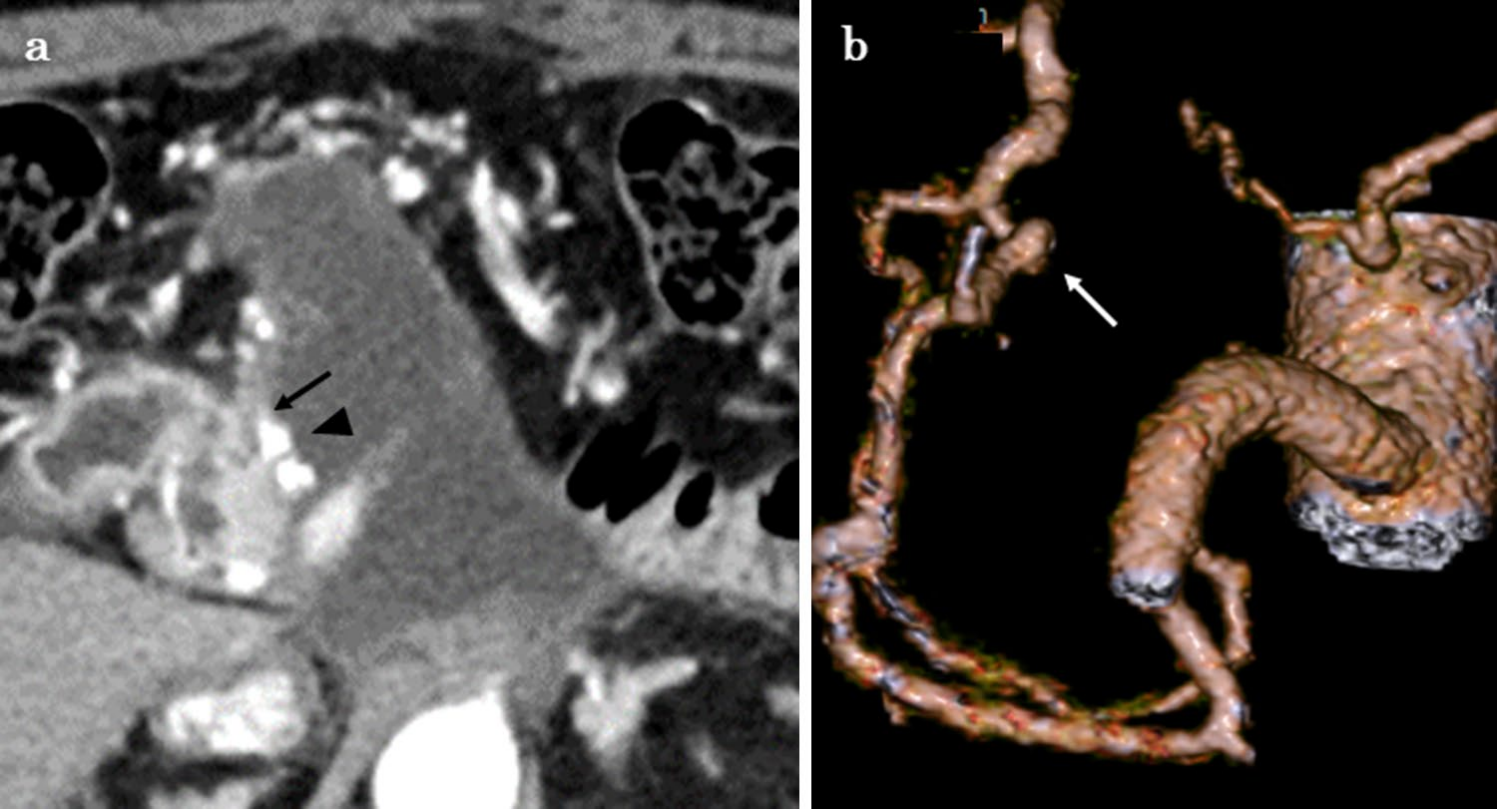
Enhanced CT findings three months after surgery. a: Enhanced CT image revealed a pseudoaneurysm (black arrowhead), 3 mm in diameter, arising from ASPDA (black arrow). Fluid collection was also observed near the pancreatic stump. b: Three-dimensional reconstruction image of the blood vessels revealed an aneurysm in the ASPDA (white arrow). *ASPDA* anterior superior pancreaticoduodenal artery, *CT* computed tomography
